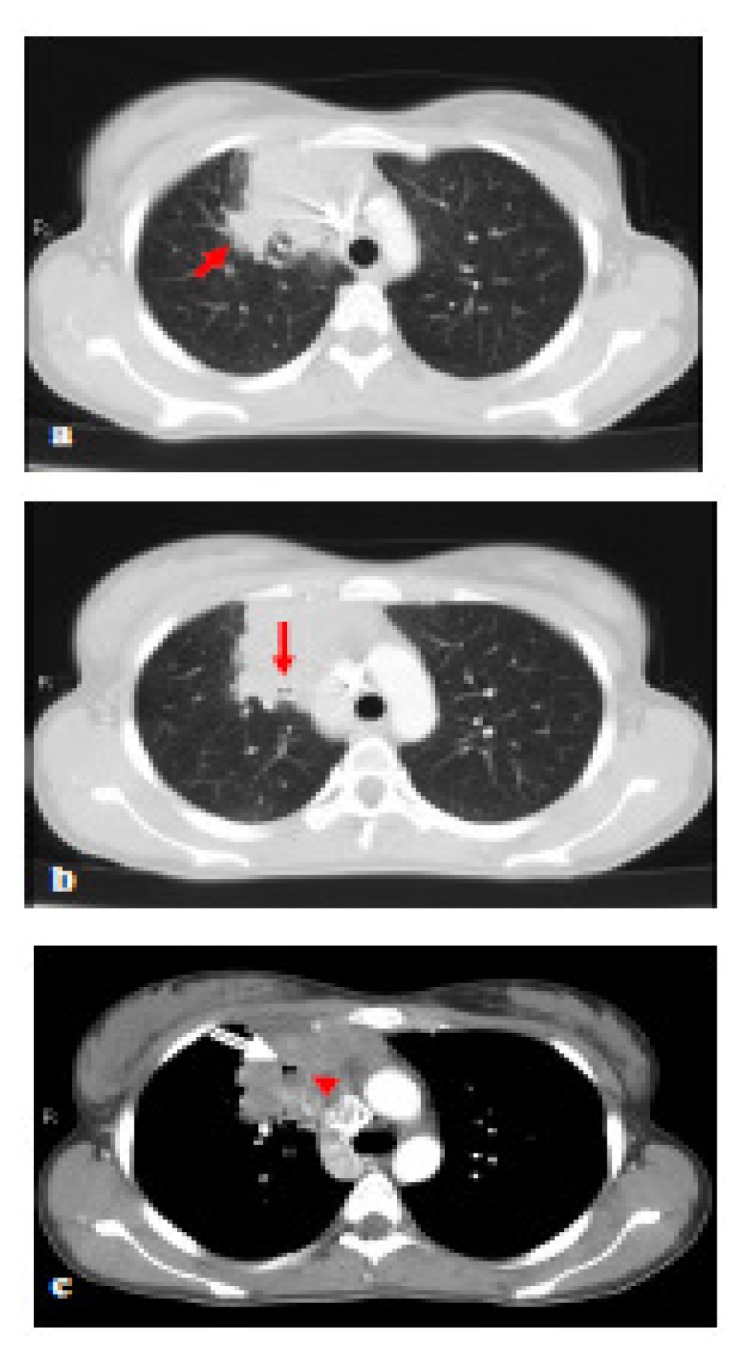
Chest CT scan at onset. A. Parenchymal lung involvement by pathologic tissue (Hodgkin’s lymphoma). The pulmonary origin is demonstrated by the irregular margins of the lesion, especially evident in correspondence with the posterolateral portion (arrow). B. Intralesional bronchial structures (arrow). C. Window to the mediastinum. It is evident the "angiogram sign" (arrowhead) in the context of the parenchymal mass. Also here is evident an intralesional bronchial branch (arrow).
